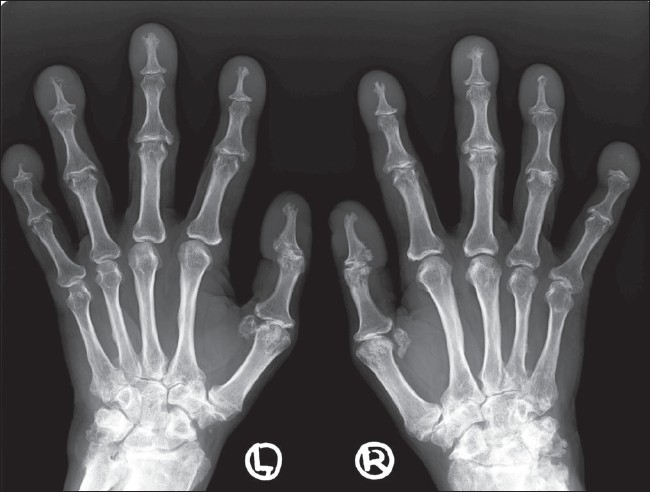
Anteroposterior radiograph of both hands shows enlargement of the distal fingers with acroosteolysis, along with modeling deformities of the sesamoid bones, reduced joint spaces at the carpus and the proximal and distal interphalangeal joints, periarticular osteopenia, and joint deformities
